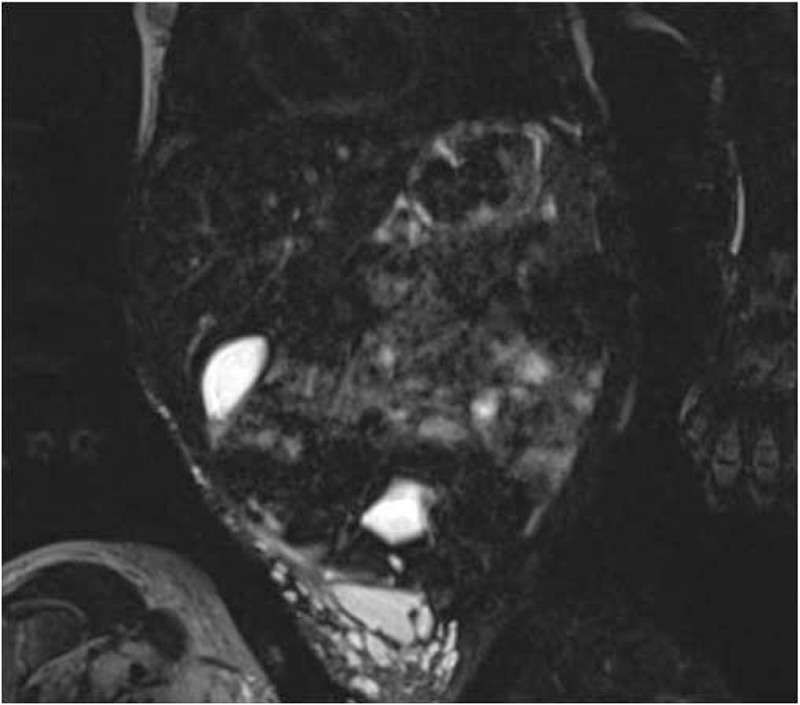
MRI scan with contrast in the coronal plane. Cysts can be observed in the subcutaneous adipose tissue of the right lower abdomen and right thigh. MRI = magnetic resonance imaging.
